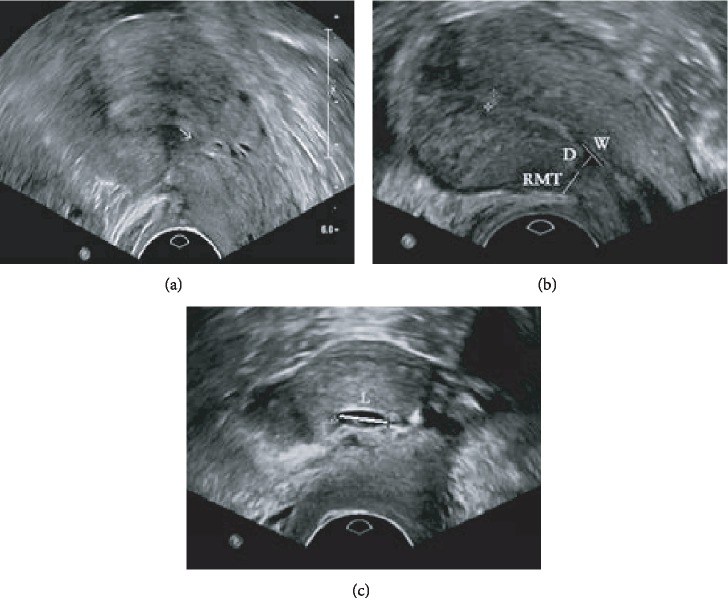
Ultrasonographic imaging of cesarean scar diverticulum (CSD) after transvaginal repair surgery. (a) Ultrasound imaging of CSD disappearance on the sagittal plane. No defect is seen in the scar shown by the arrow. (b) Ultrasound imaging showing CSD remained on the sagittal plane. (c) Ultrasound imaging showing CSD remained on the transverse plane. *W*: width of the scar niche on the sagittal plane; *D*: depth of the scar niche on the sagittal plane; RMT: residual myometrial thickness on the sagittal plane; *L*: length of the scar niche on the transverse plane.
